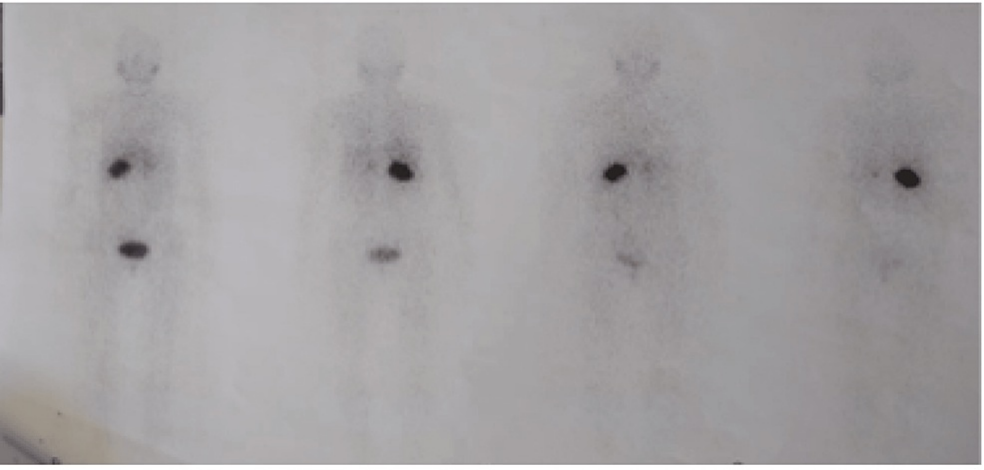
MIBG scintigraphy showing fixation opposite both adrenals MIBG: meta-iodobenzylguanidine
